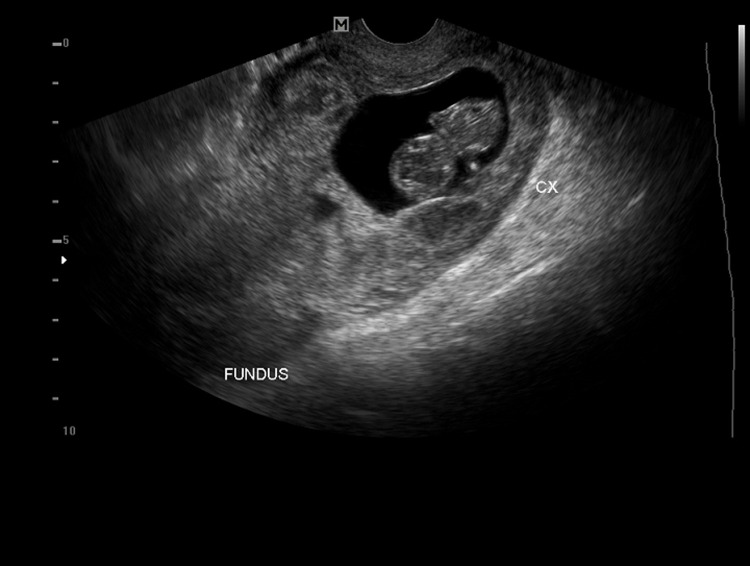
transvaginal ultrasound image of the uterus and cervix; the image shows an empty uterus; a gestational sac with a fetal pole is located in the endocervical canal
